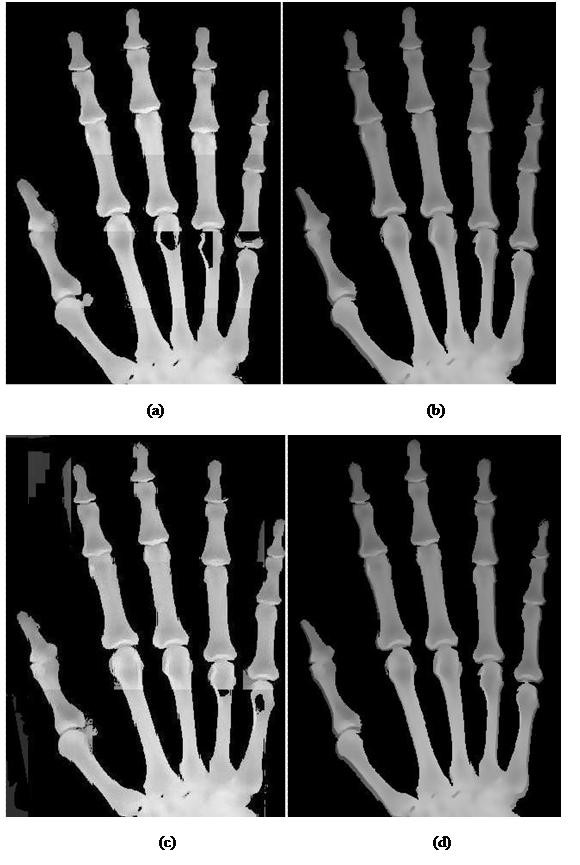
Comparison of hand radiograph before and after the BAE algorithms. In this figure, the image presented are (a) Hand radiograph before BAE (b) Recovery of the artifacts of finger radiograph (c) Hand radiograph with anomalies and noise (d) Finger radiograph free from anomalies and noise after BAE.
